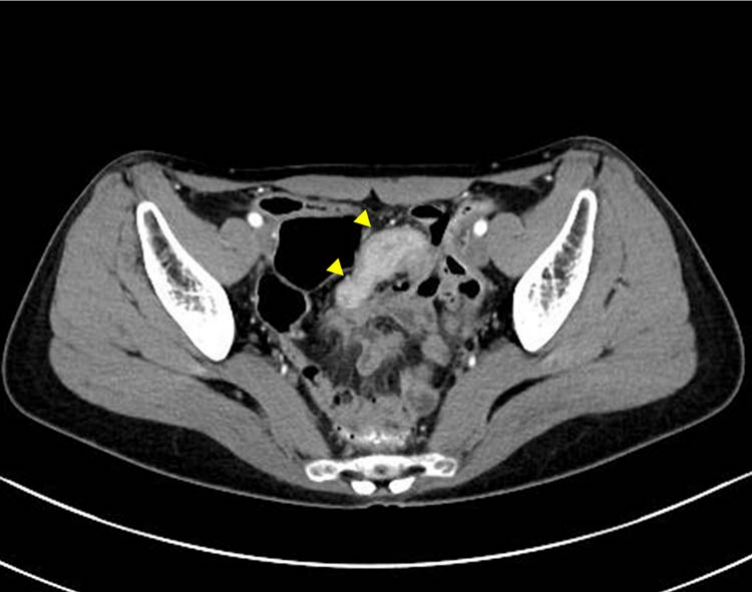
Computed tomographic scan showing a continuous lesion to the ileum with contrast enhancement (arrowheads).
